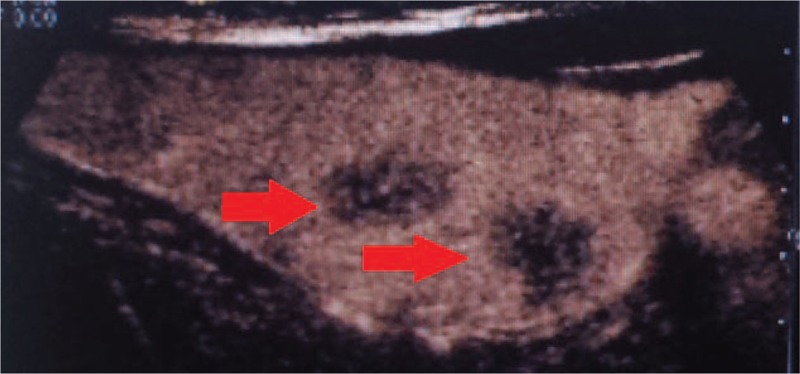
Thyroid ultrasound image: Sulfur hexafluoride contrast-enhanced ultrasound revealed nodular goitre with no malignant changes. Two thyroid nodules (above: 21 mm × 11 mm; below: 14 mm × 21 mm) were represented by red arrows.
